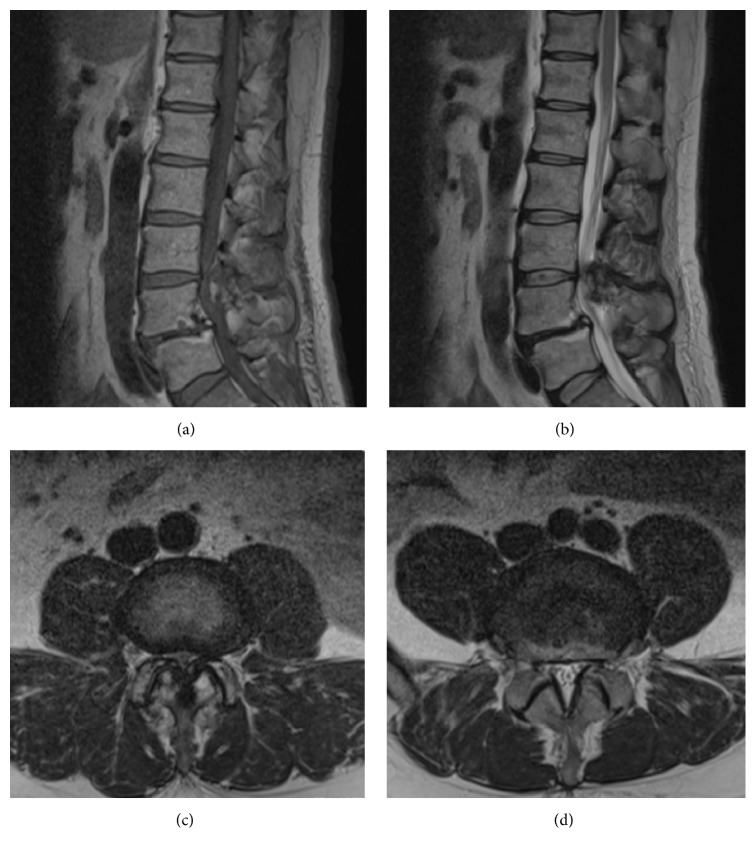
Preoperative magnetic resonance imaging. Sagittal T1-weighted image (a); sagittal T2-weighted image at the midspinal canal (b); axial T2-weighted image at the L3/4 level (c); axial T2-weighted image at the L4/5 level (d). Lumbar canal stenosis is seen at the L3/4 and L4/5 levels, with severe stenosis at L3/4.
